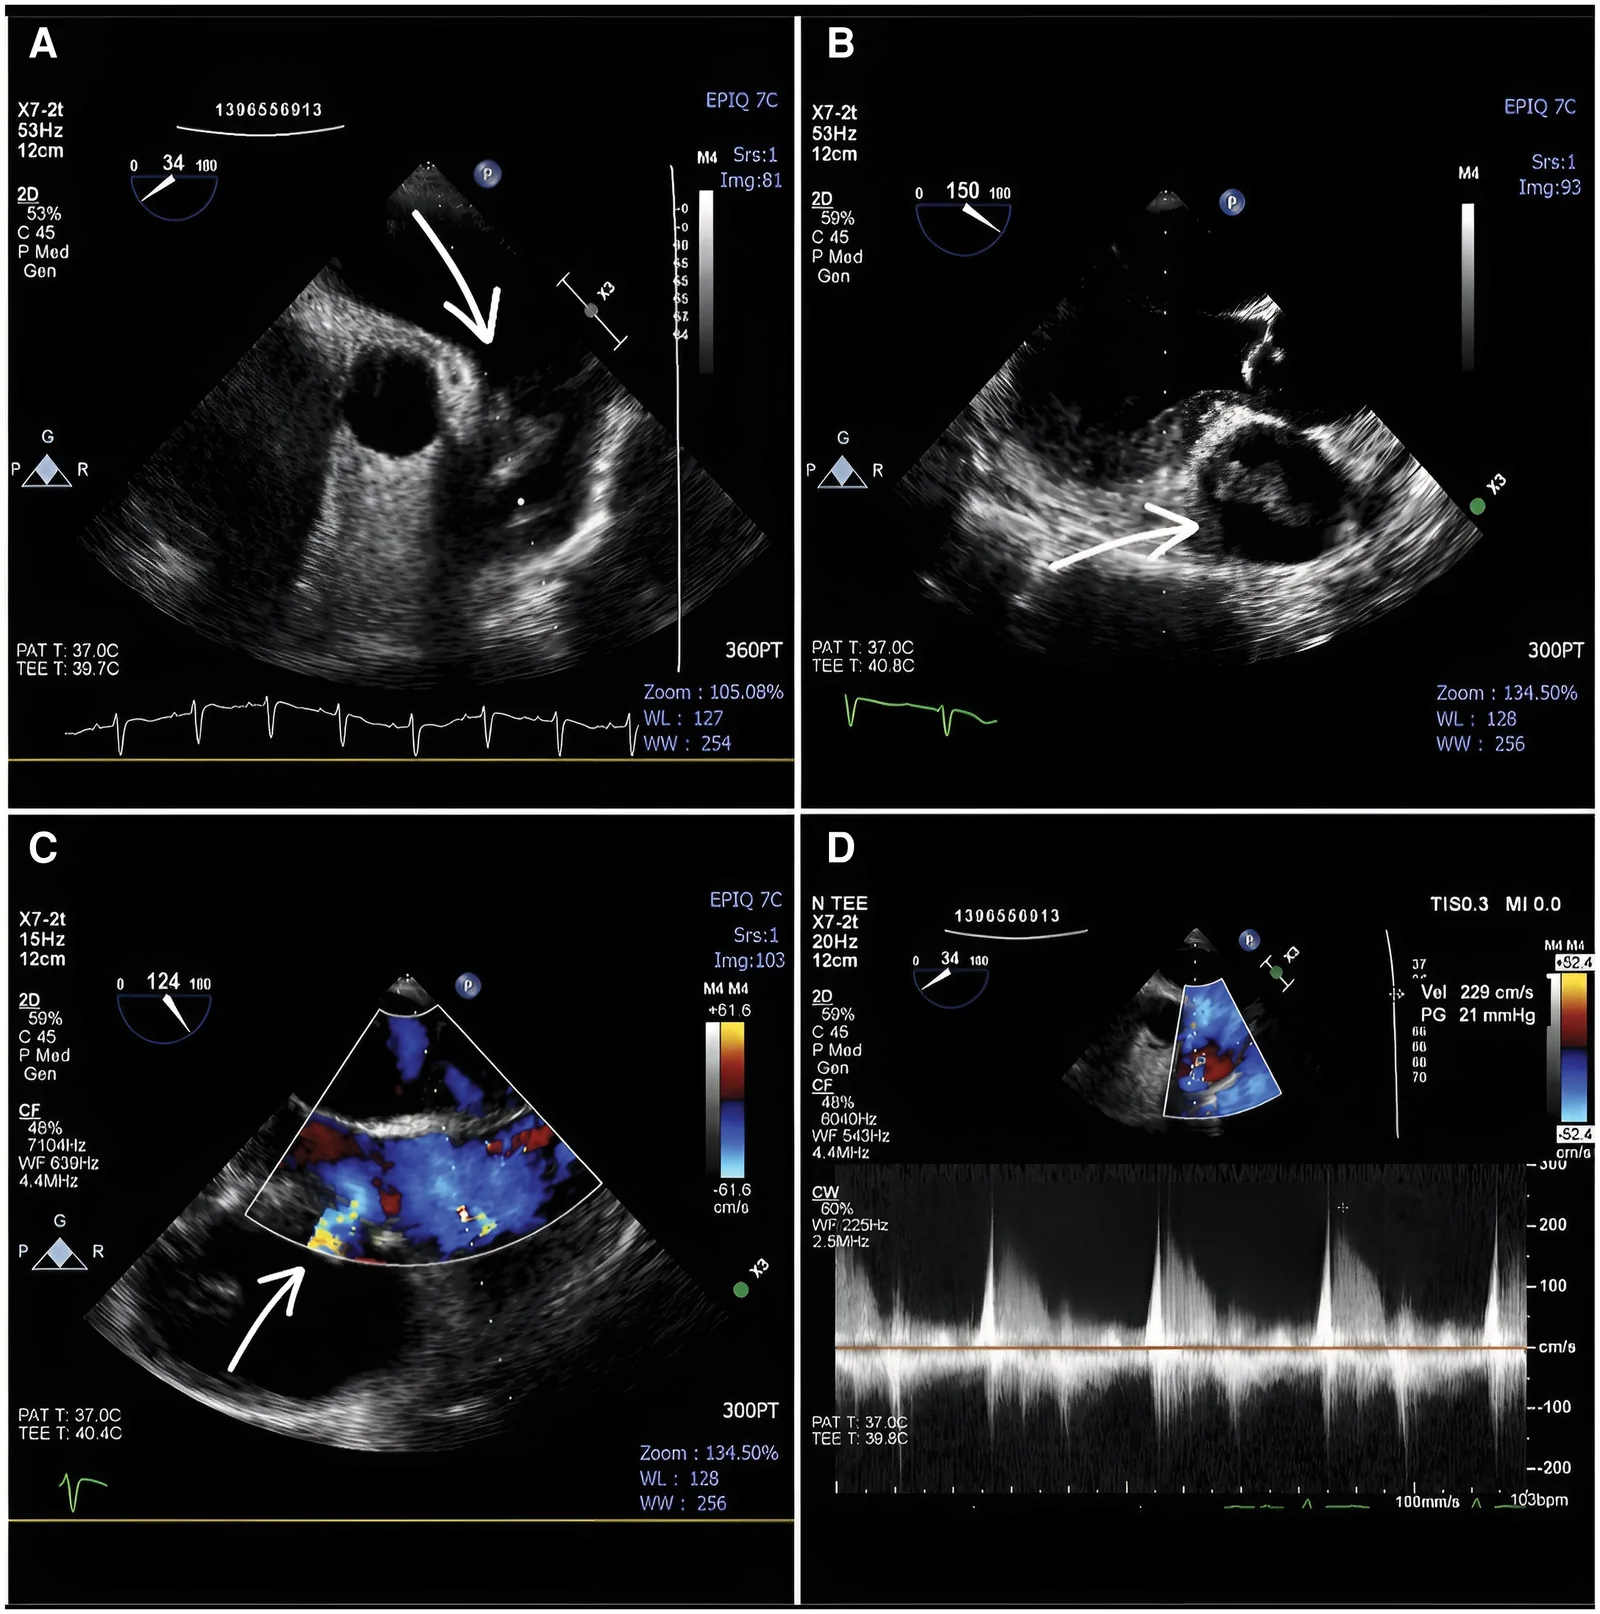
Transoesophageal echocardiography (TEE) demonstrating pulmonary valve involvement. (*A*) Mid-oesophageal TEE right ventricular outflow tract (RVOT) view showing a mobile echo-dense mass attached to the pulmonary valve, consistent with vegetation. (*B*) Additional TEE off-axis view confirming the presence and mobility of the pulmonary valve vegetation. (*C*) Ventricular septal defect (VSD) with left to right shunt. (*D*) Doppler study of moderate pulmonary insufficiency (PI).
